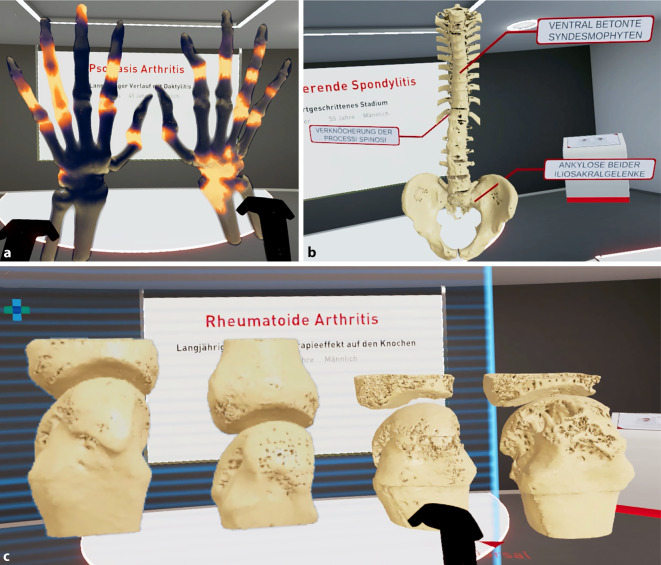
In dieser Form ist das Blockpraktikum auf einen Einsatz für Medizinstudierende im 9./10. Semester (5./6. klinisches Semester) ausgerichtet. Die Evaluation der Module erfolgte mittels eines Fragebogens unmittelbar nach dem Praktikum und umfasste geschlossene Fragen zur subjektiv empfundenen Wirksamkeit mit der Wissensvermittlung innerhalb der einzelnen Module unter Zuhilfenahme der Likert-Skala mit den Ausprägungen: sehr wirksam, eher wirksam, mäßig wirksam, neutral oder nicht wirksam. Die an diesem Blockpraktikumstag erlernten Fähigkeiten zur Anamnese sowie zu körperlichen und sonographischen Untersuchungen wurden in den darauffolgenden Tagen der Praktikumswoche im Rahmen der ambulanten Sprechstunde und der Stationsvisite anhand realer Patientinnen und Patienten vertieft. Diese Studie wurde von der zuständigen Ethik-Kommission der FAU Erlangen-Nürnberg genehmigt (Votum Nr. 302_20 Bc).
